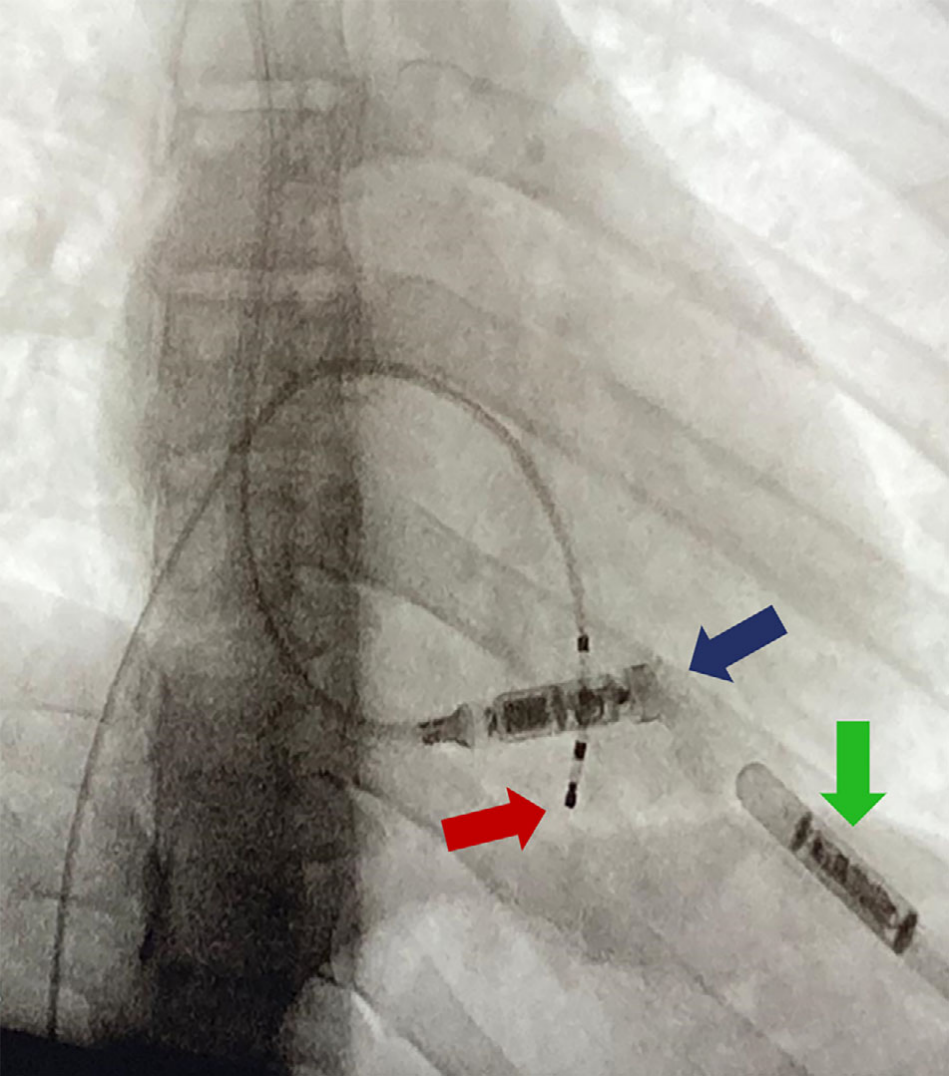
Radiographic image showing the leadless pacemaker delivery catheter and the pacemaker in situ (blue arrow), demonstrating accurate placement within the target cardiac structure. The red arrow indicates the quadripolar temporary pacing catheter, and the green arrow identifies the implantable loop recorder. [Colour figure can be viewed at wileyonlinelibrary.com]
